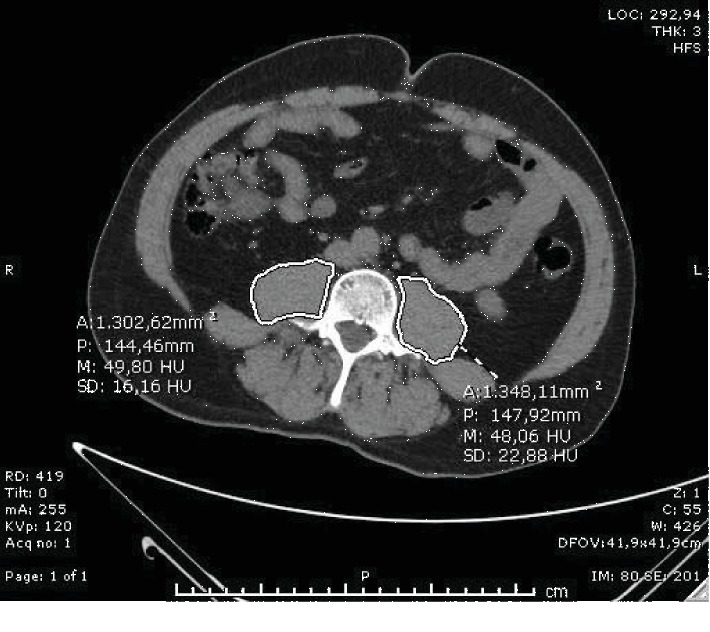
43-year-old man with pulmonary embolism managed in intensive care unit. The value of psoas muscle area was obtained by dividing the sum of the right and left psoas muscle areas at the level of L3 vertebrae into body surface areas using single axial CT scan images. He had lower PESI scores, high arterial oxygen saturation, and lower respiratory rate without in-hospital mortality.
